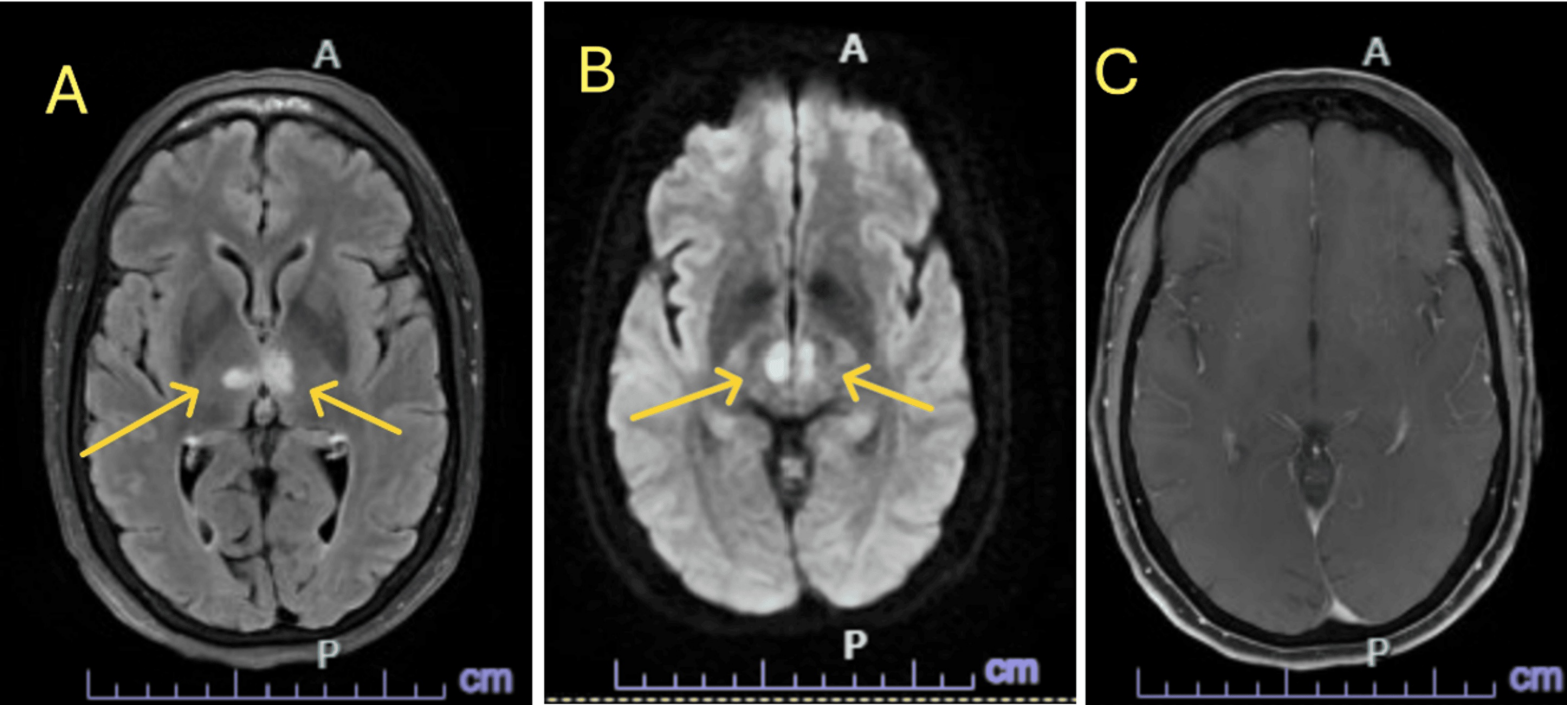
MRI Brain axial view. T2 FLAIR Propeller (A). DWI (B). T1 SPGR FS +C (C). This image shows bilateral medial thalamic diffusion restriction and edema without associated enhancement, consistent with AOP territory infarction and raised this differential diagnosis to the top position. Cytotoxic edema, characterized by restricted diffusion, reflects swelling of individual cells due to impaired cellular function. MRI: magnetic resonance imaging, Ax: axial, T1: spin-lattice relaxation time, SPGR: spoiled gradient recalled, FS: fat saturation, +C: with contrast, FLAIR: fluid-attenuated inversion recovery, DWI: diffusion-weighted imaging, AOP: artery of Percheron
